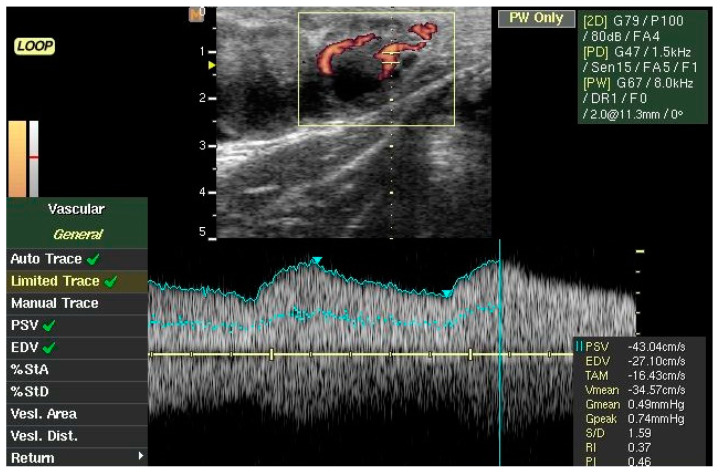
Corpus luteum blood flow assessment in buffaloes with the aid of eco-color Doppler mode.
